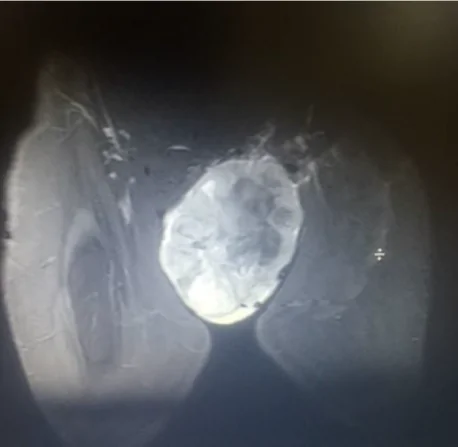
MR image of the mass in the vulva
